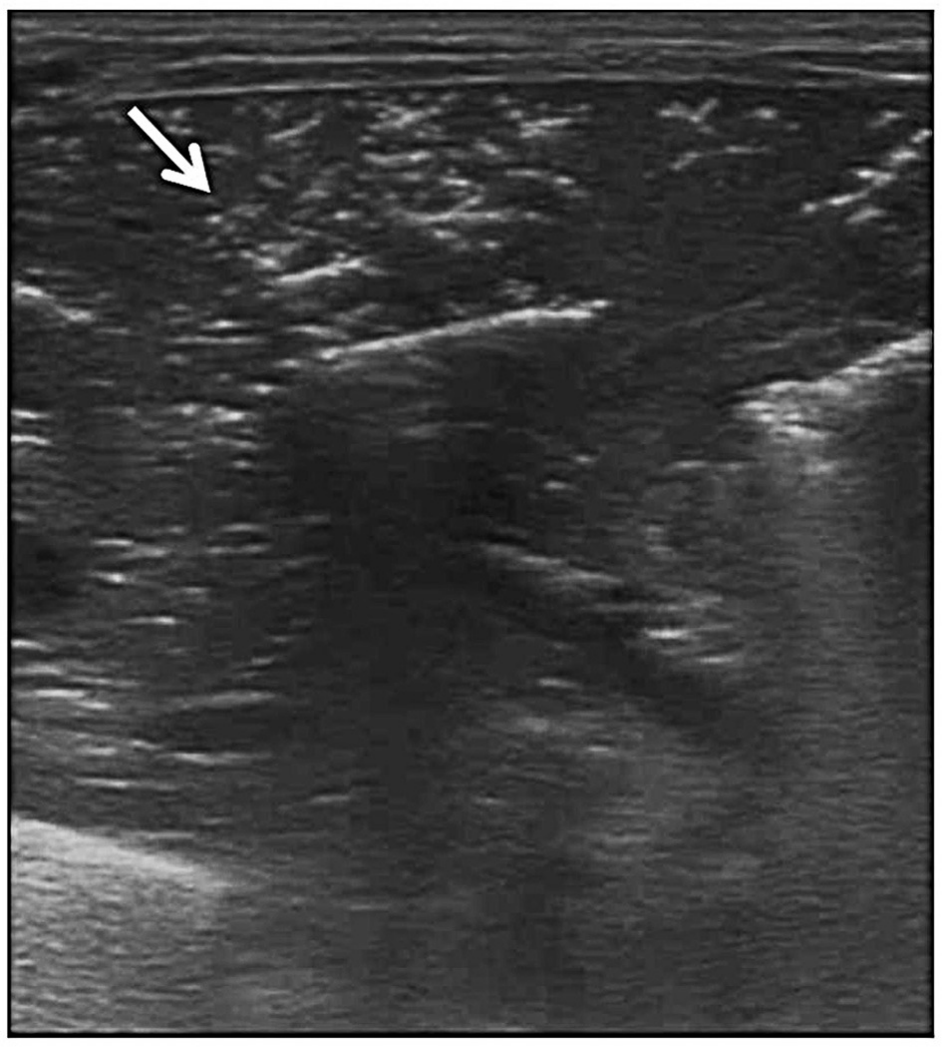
Portal venous gas. Grayscale ultrasound shows portal venous gas from the small intestine (arrow).
